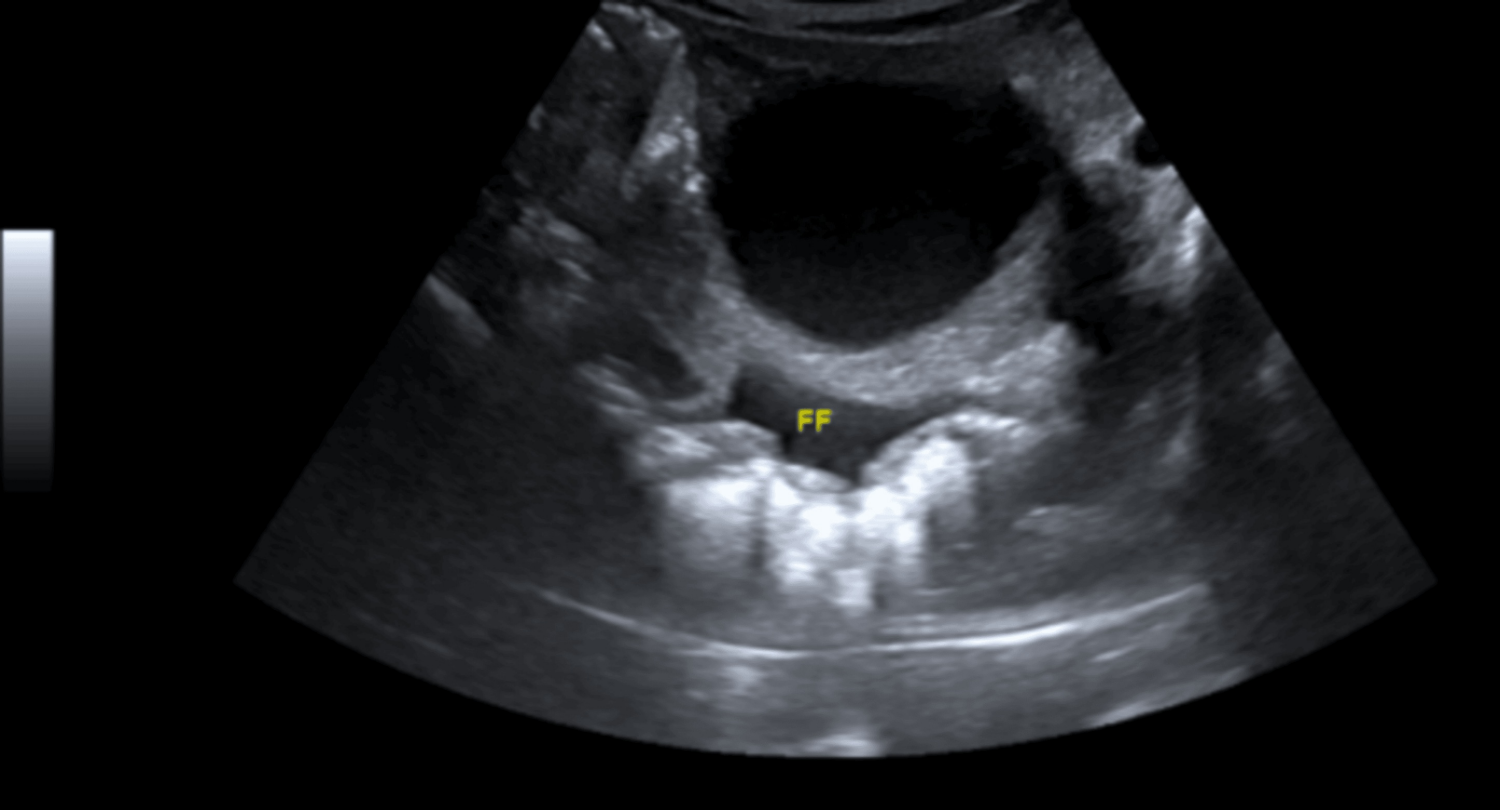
Another view of abdominal ultrasound showing free turbid peritoneal fluid
